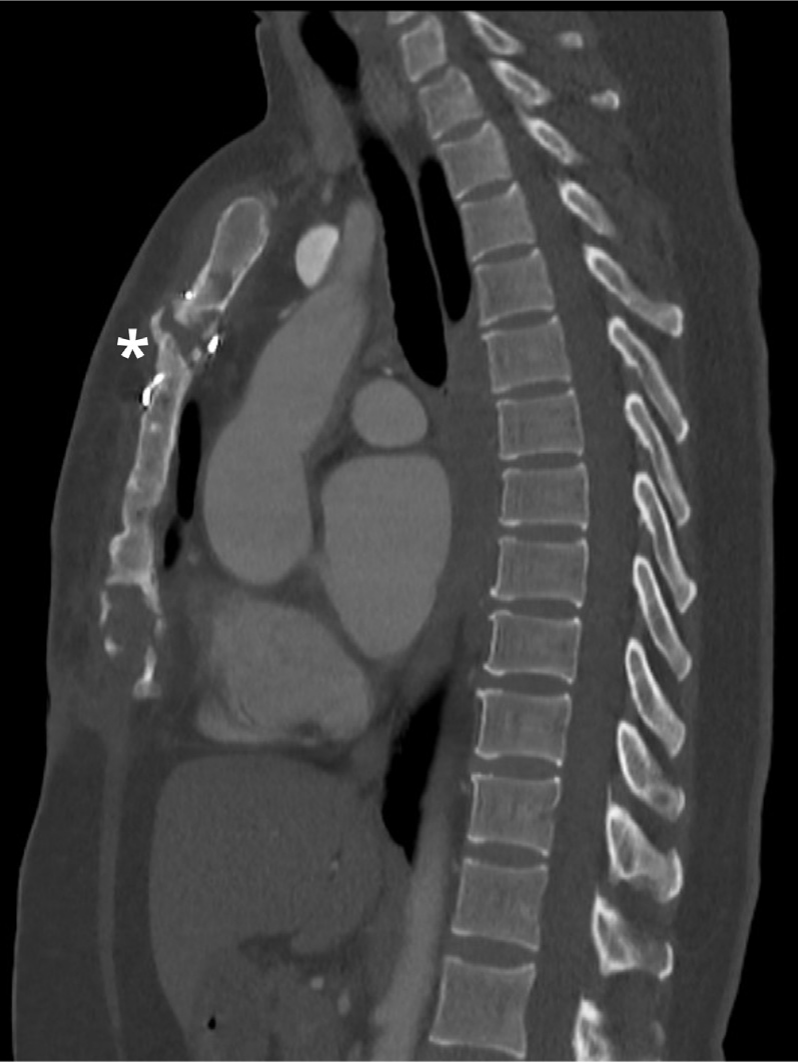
Chest Computed Tomography With Signs of Sternal Osteomyelitis Scan showing corticomedullary chronic sclerotic inflammatory signs at the body-manubrium sternal union (asterisk).
